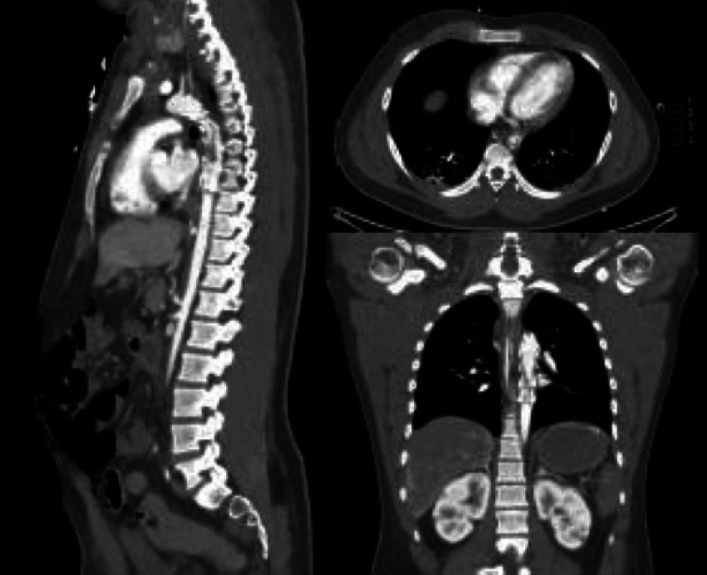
CT scan day 1 postendovascular TPA and angioplasty. An intraluminal filling defect that extend from the proximal descending aorta to the distal tip of the stent graft. There is disappearance of the large bulk of the in-stent thrombus with residual cord-like fibrous components. Abdominal aorta and its major branch vessels are severely diminutive in caliber. New extensive hepatic, renal, and splenic infarcts. Evidence of pneumatosis and hypoenhancement of the bowel.
